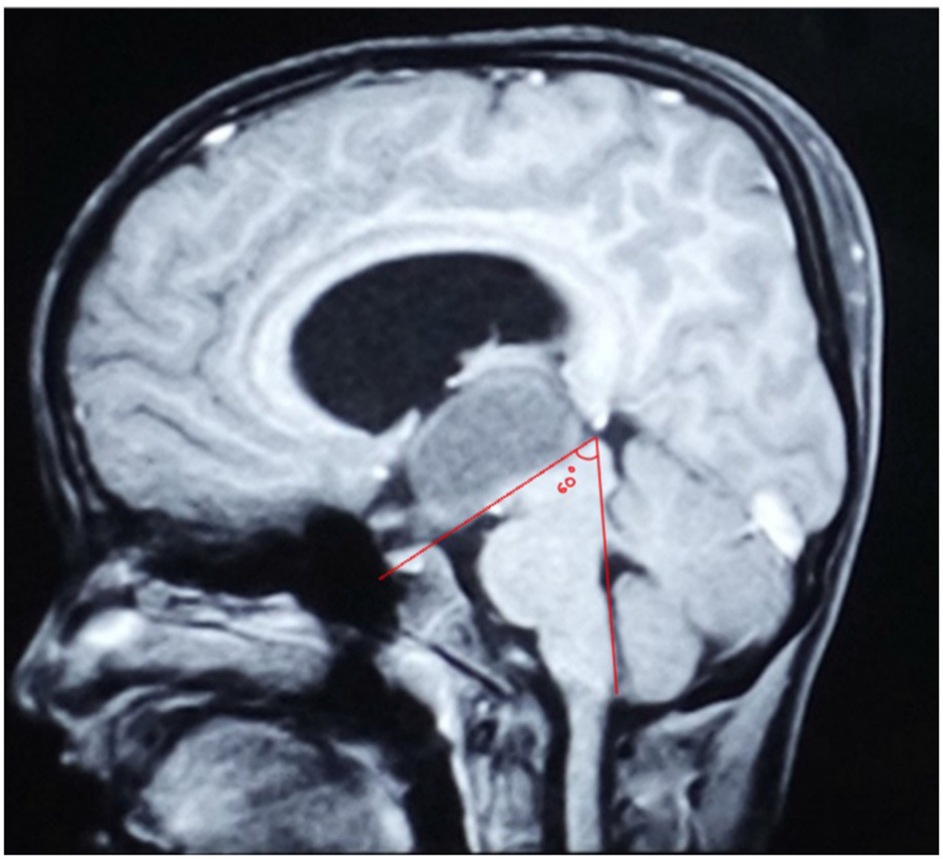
Mid-sagittal MRI image of an 8-year-old boy with a purely intraventricular craniopharyngioma showing the Mamillary Body Angle being 60 degrees.
